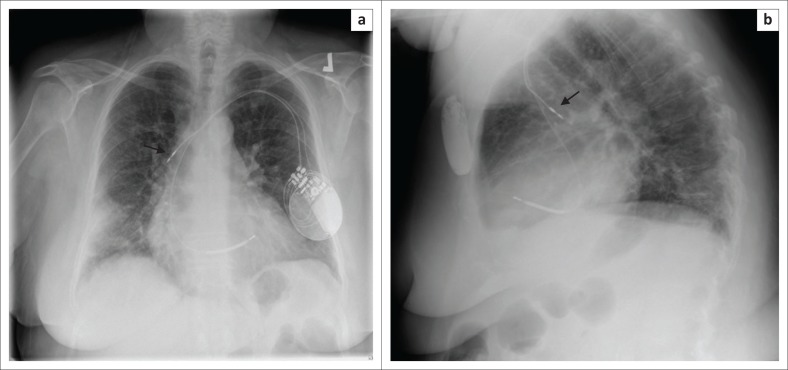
Posterior–anterior (a) and lateral (b) chest radiograph views showing a dual-lead implantable cardioverter defibrillator with a single right ventricular shock coil. Note its right atrial lead (arrow) malpositioned in the superior vena cava.
